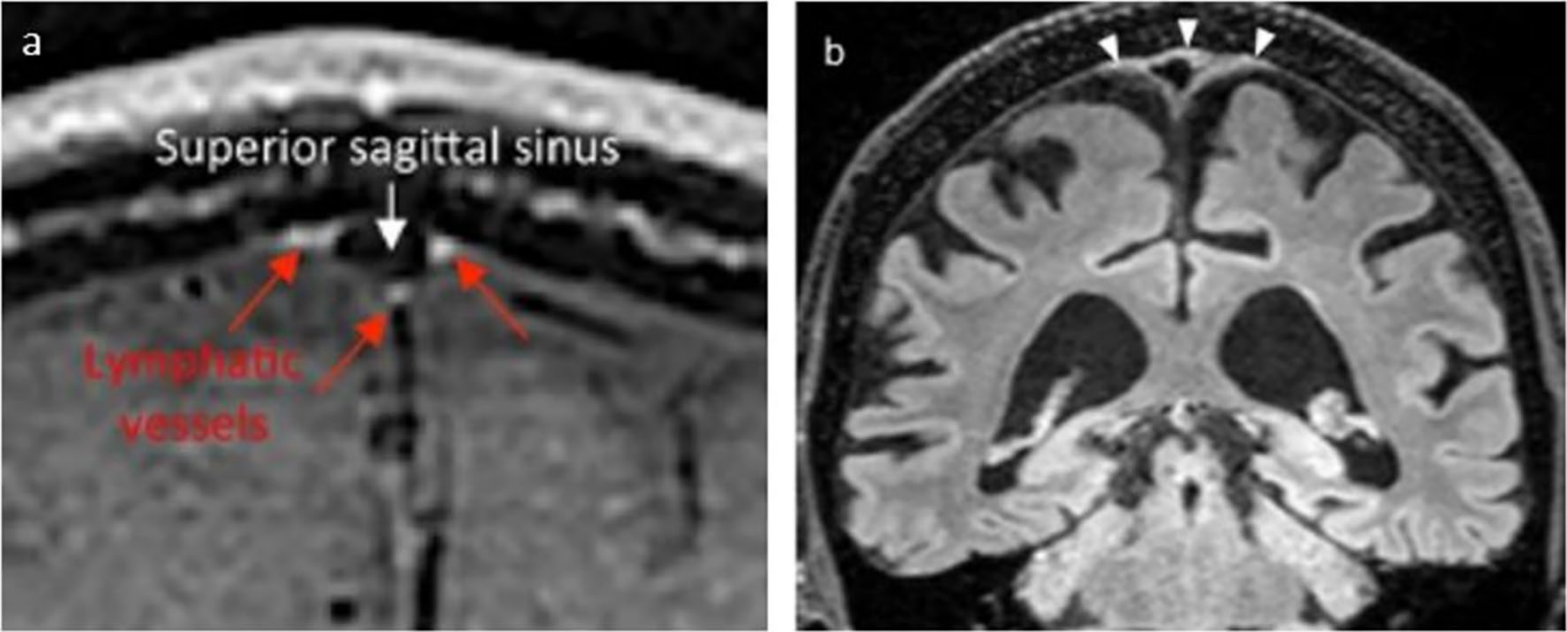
Examples of interpretation of lymphatic tissue in parasagittal dura. In a intravenous contrast enhancement in parasagittal dura is interpreted as lymphatic vessels (red arrows), while blood flow in superior sagittal sinus is suppressed (From Absinta et al. eLife 2017) [37]. In b all hyperintense FLAIR-signal in parasagittal dura (arrowheads) was accepted as lymph (From Albayram et al. Nature Communications, 2022) [54]. Figure elements are reused with permission under the Creative Commons International License
